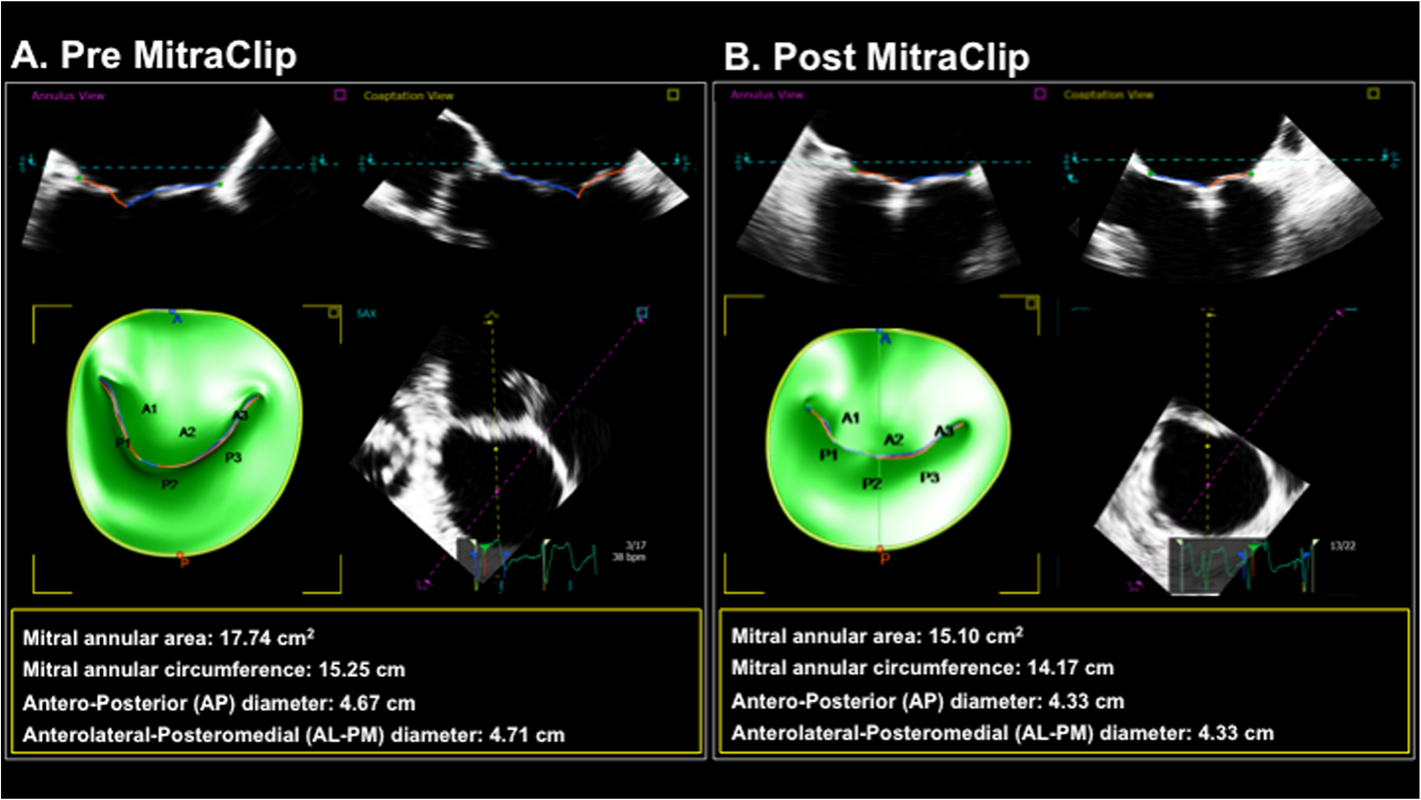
MitraClip Induced Mitral Annular Remodeling on 3D Transesophageal Echo. Representative example of mitral apparatus remodeling parameters as quantified using intra-procedural 3D TEE. Note device-induced reductions in mitral annular circumference and area, paralleling post-procedure reductions in mitral annular linear indices
